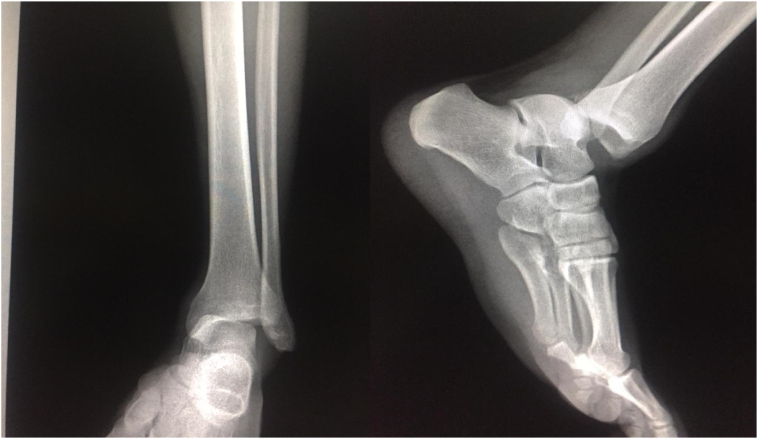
Standard front and side radiograph showing pure posteromedial tibiotal dislocation.
